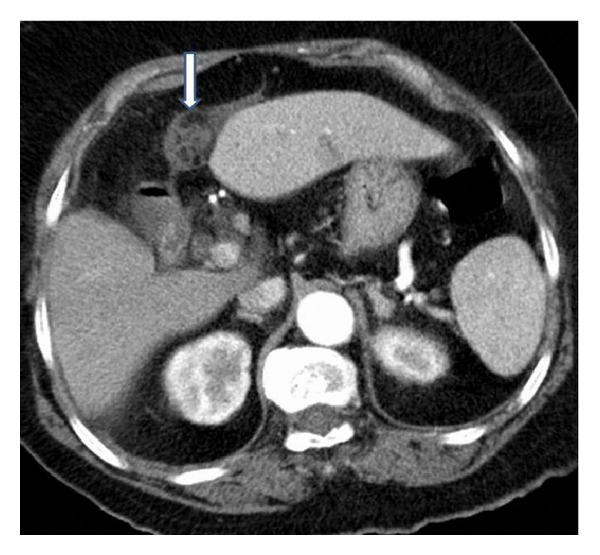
An abdominal computed tomography scan showing a mass lesion (arrow) originating from the gallbladder region extending to the periportal region and surrounding the falciform ligament with air density and reactionary fluid.
